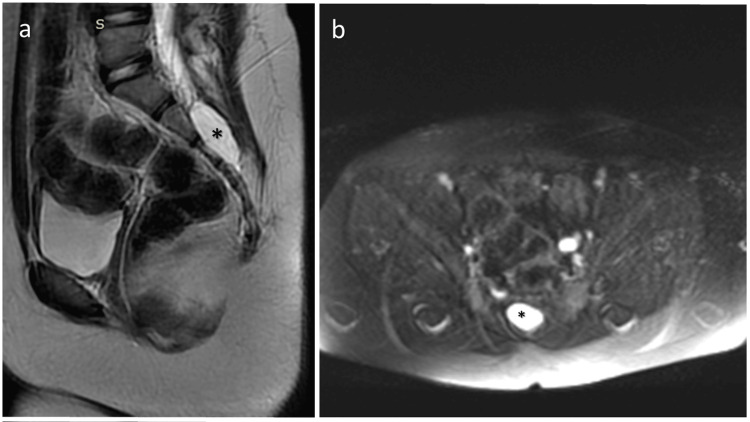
Sagittal T2 (a) and axial fat-suppressed T2 (b) MRI images showing a thin-walled Tarlov cyst measuring 43 x 17 x 23 mm located at the level of the S2-S4 vertebral corpus within the spinal canal and slightly to the right of the midline MRI: magnetic resonance imaging
